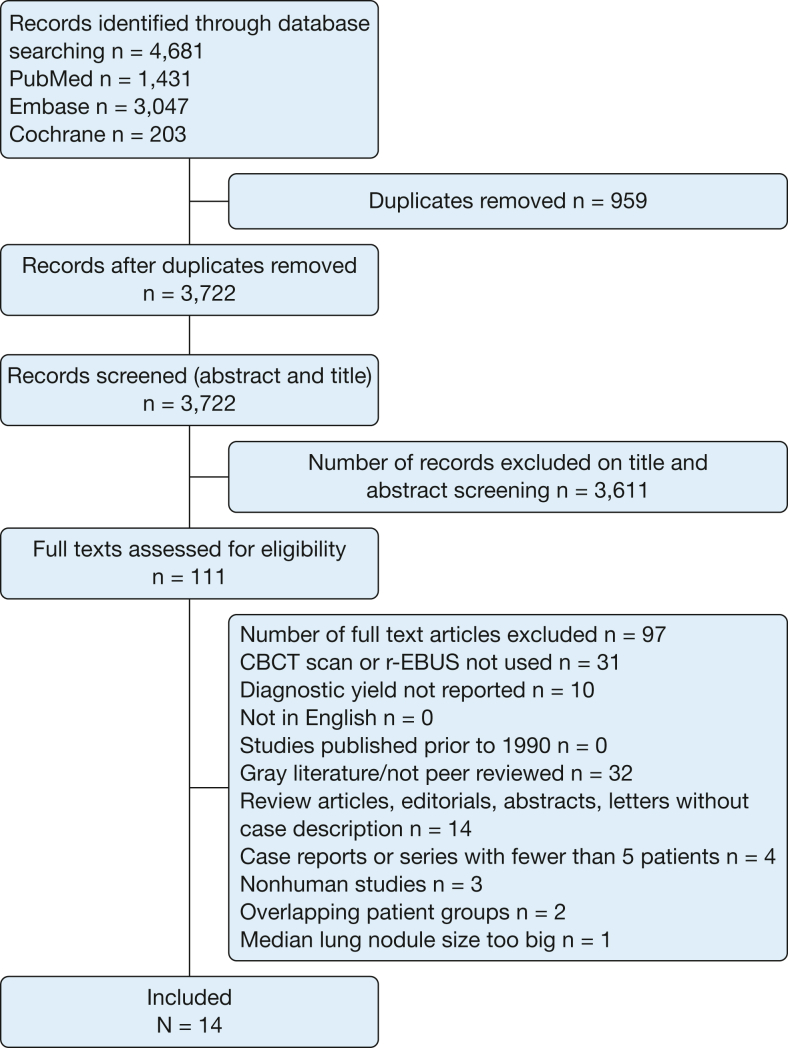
Systematic review method. CBCT = cone beam CT; r-EBUS = radial endobronchial ultrasound.
